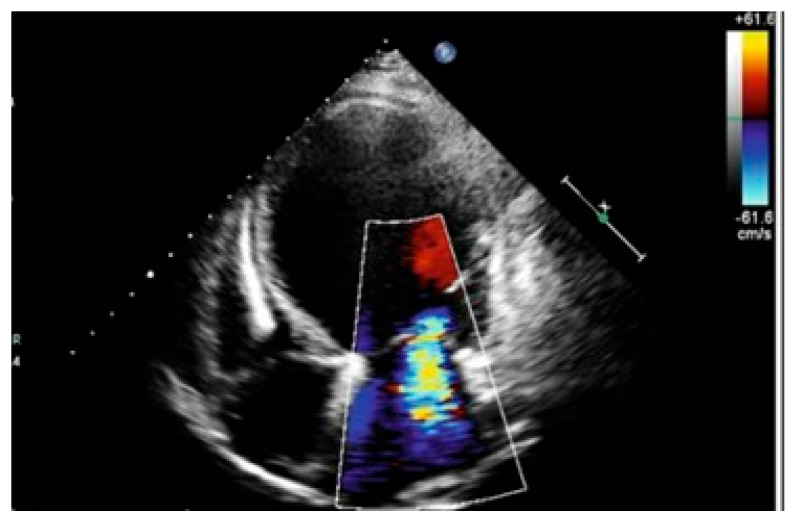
Echocardiographic findings (apical four-chamber view) at admission that revealed a grade III mitral regurgitation and mildly dilated left ventricle. The color scale reveals red tones for the blood approaching the transducer and blue tones for the blood moving away from the transducer.
